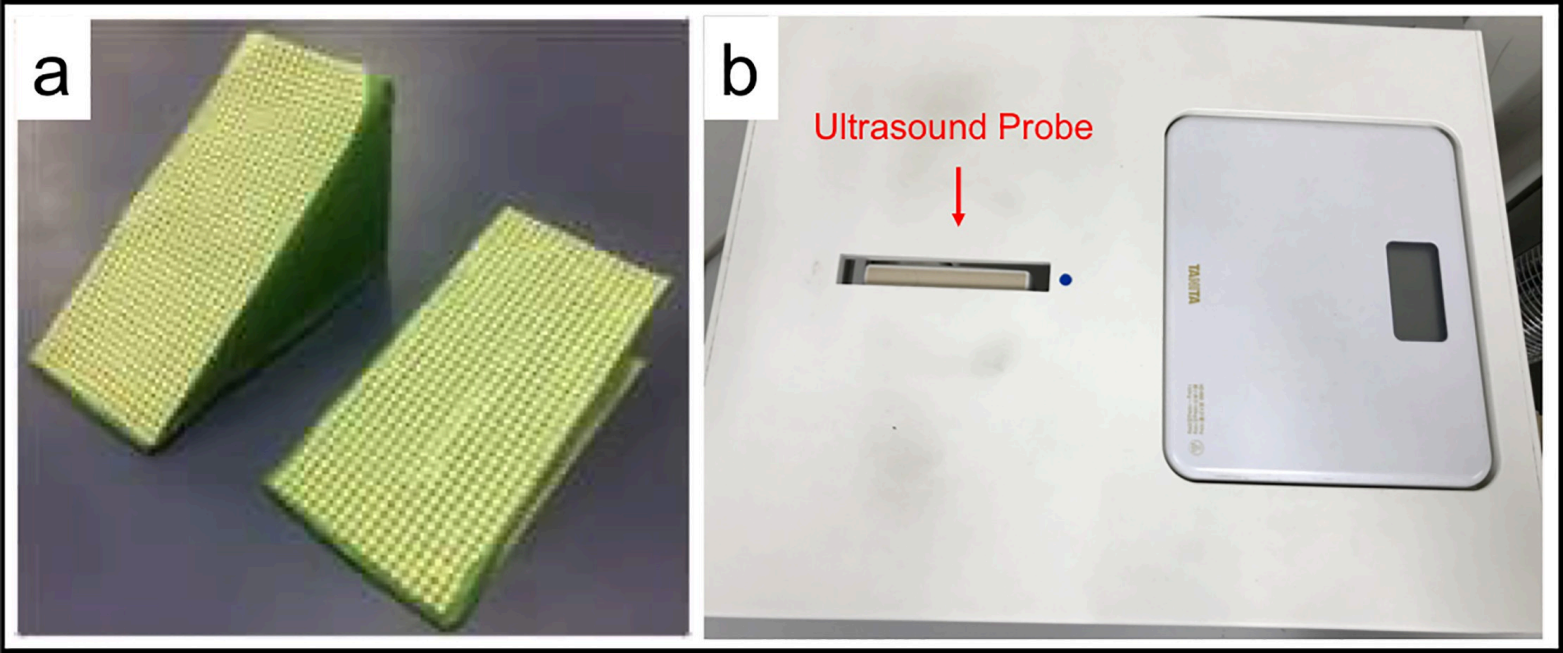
Measurement equipment. a) two custom-made high heels of 15 degrees inclination and 30 degrees inclination; b) WPUID containing an ultrasound probe and a weight scale, allows to take coronal views of the transverse arch of the foot.
